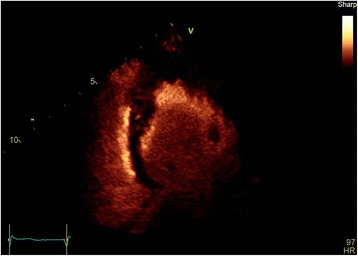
Apical four-chamber contrast echocardiography showing apical left ventricular hypertrophy
